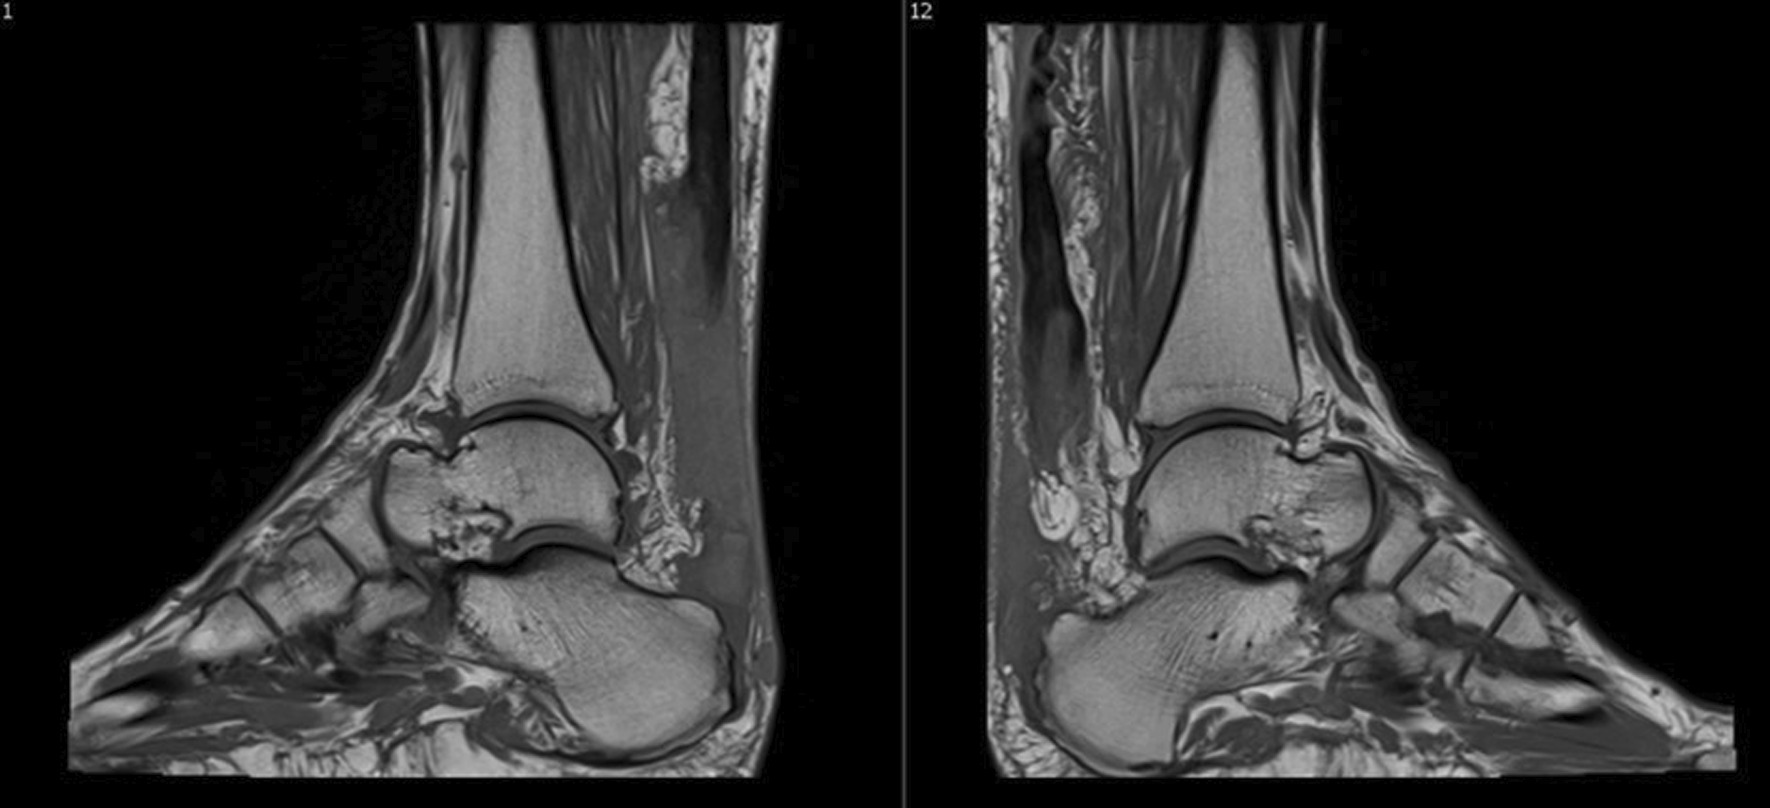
Magnetic resonance imaging showing complete avulsion of the Achilles tendons from calcaneus insertions
